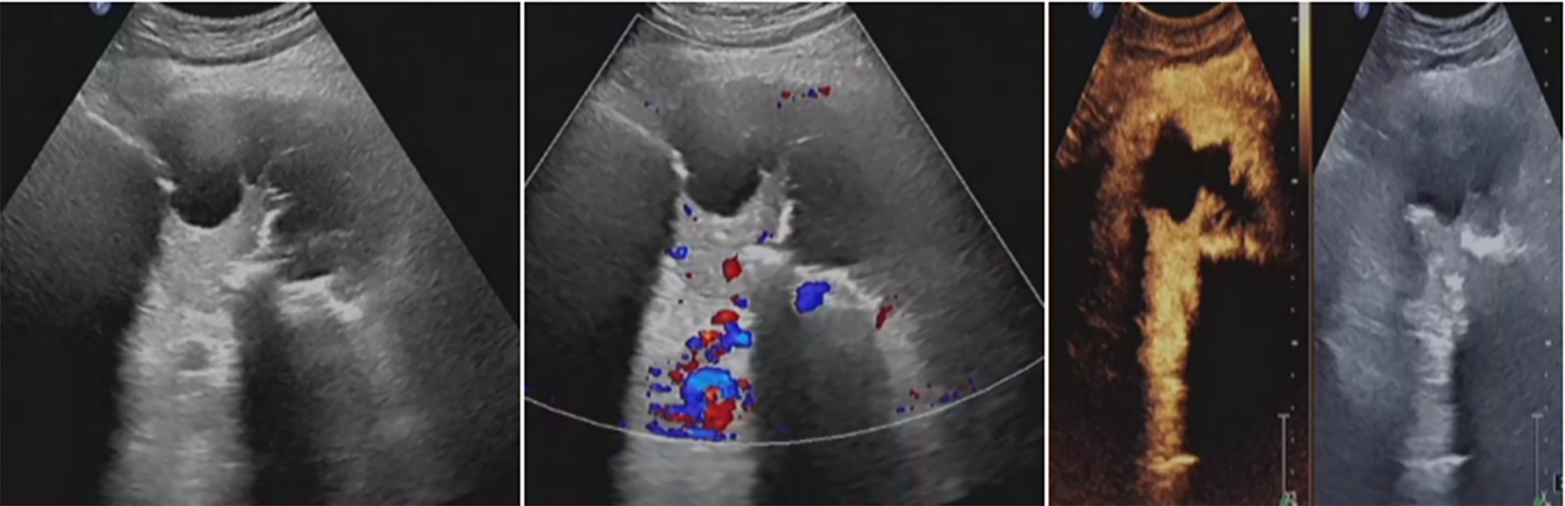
Residual tumor was inactivated by ultrasound-guided fractionated radiofrequency ablation. The ablation range was preliminarily estimated to reach 90%, achieving the expected therapeutic goal.
